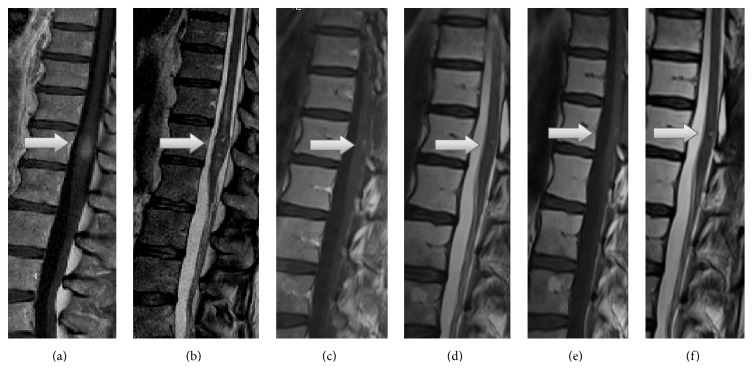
Preoperative sagittal MRI shows the spinal cord tumor at the level of the conus medullaris (arrow). T1-weighted MRI with gadolinium shows high signal intensity relative to that of the cord (a) and T2-weighted MRI shows inhomogenous hypointensive signal (b). Four weeks' follow-up sagittal T1-weighted MRI with gadolinium (c) and T2-weighted MRI (d) and 28 weeks' follow-up T1-weighted MRI with gadolinium (e) and T2-weighted MRI (f) show the residual tumor (arrow).
